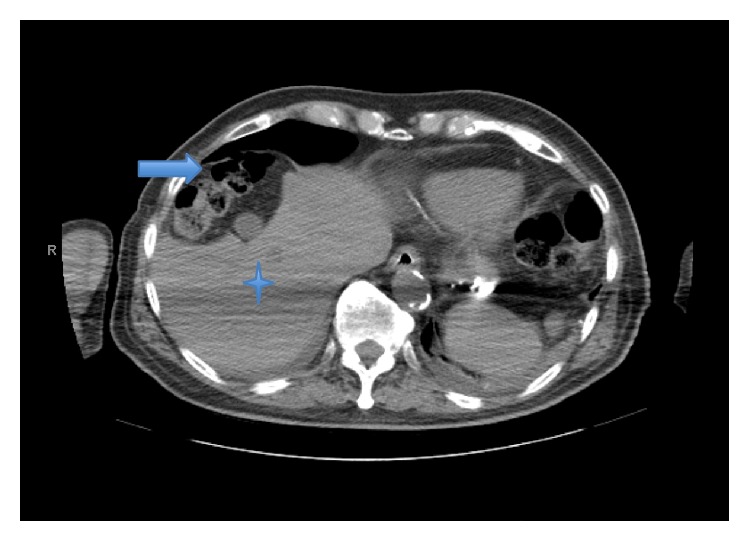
CT of the abdomen (axial section) showing a loop of colon (arrow) between the liver (asterisk) and the right hemidiaphragm.
